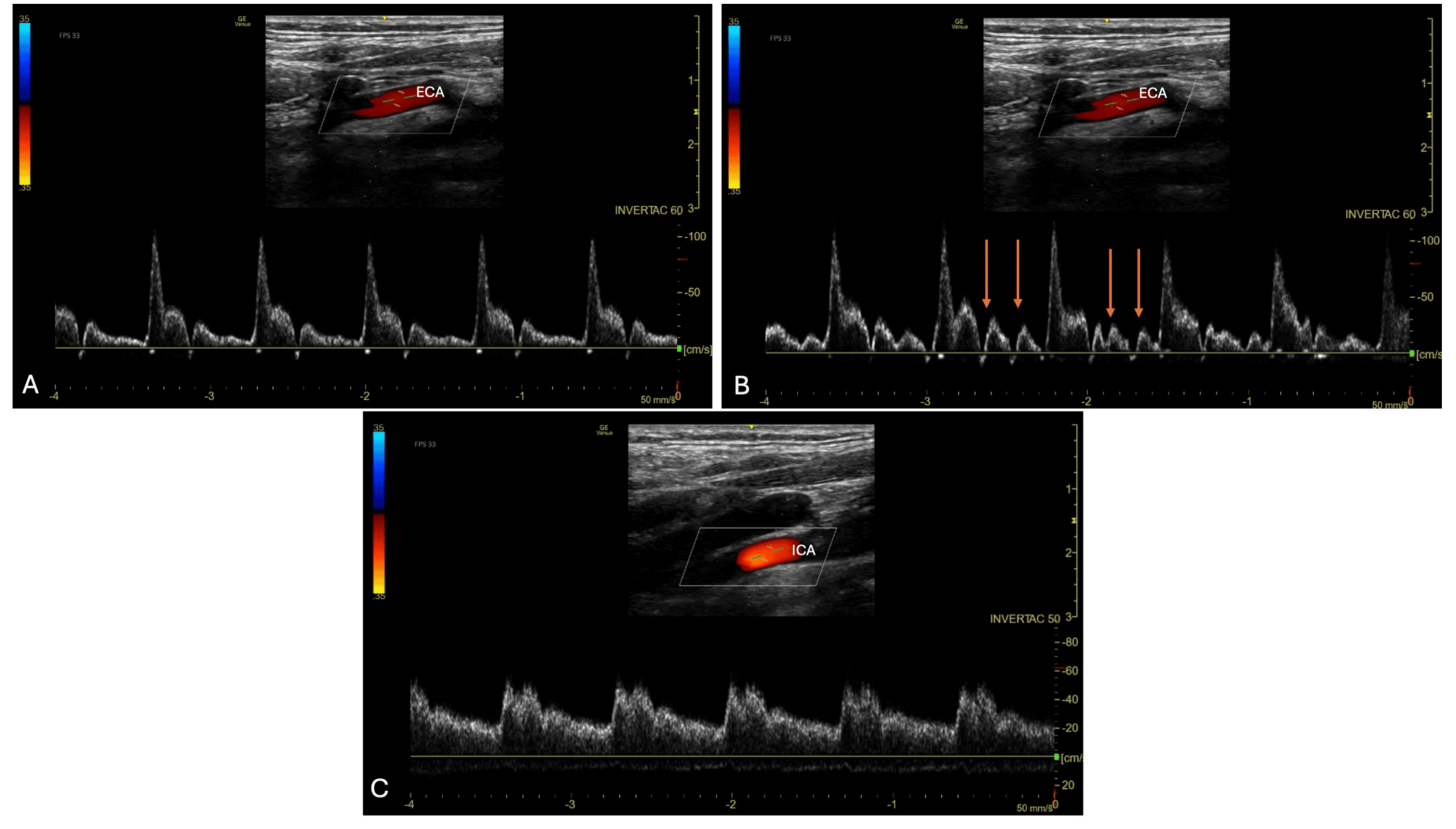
In-plane xarotid Doppler using a linear-array probe. A: External carotid artery with its higher resistance pattern. B: External carotid artery with transmitted ‘tapping’ oscillations demonstrated. C: Internal carotid artery with a lower resistance waveform pattern.
